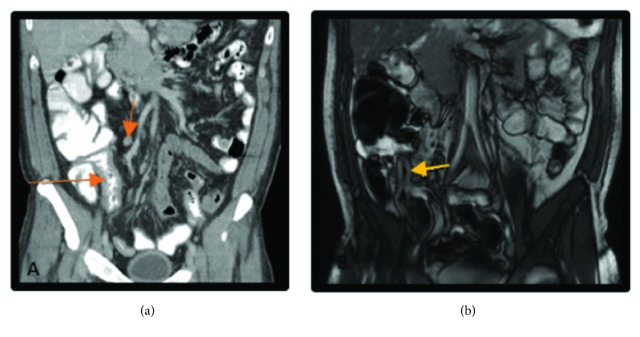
CT, MR Imaging. (a) Coronal CT, irregular wall thickening of the TI (long orange arrow), and mild RLQ lymphadenopathy (short orange arrow). (b) MRE, wall thickening of TI (yellow arrow).
